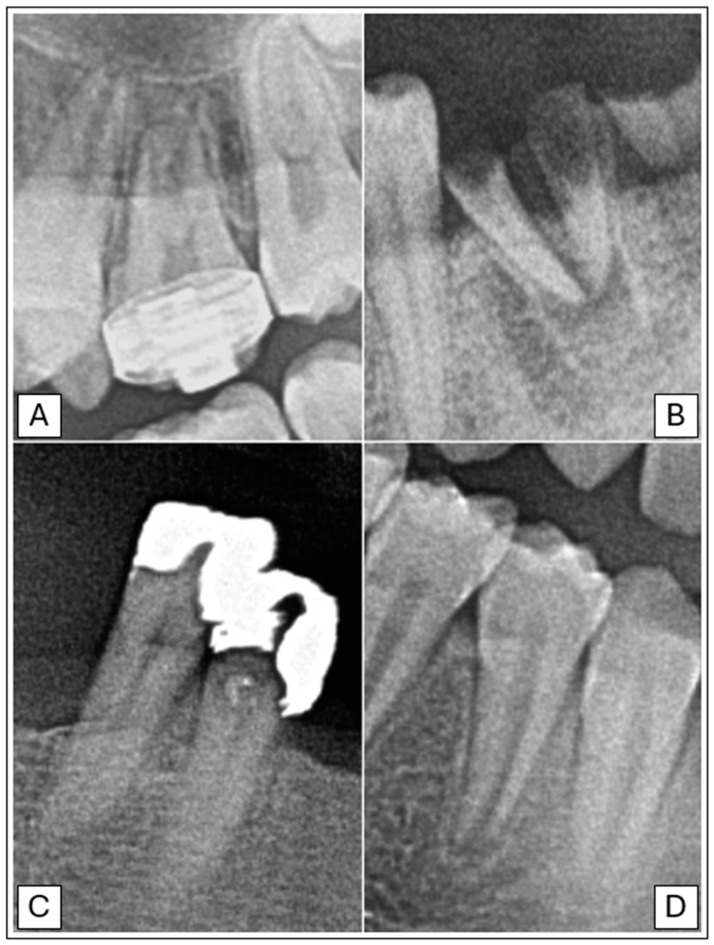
Examples of teeth misdiagnosed by AI software. (A) Tooth 26 with an orthodontic band, mistakenly identified as a tooth with a crown. (B) Tooth 36, not treated endodontically, incorrectly diagnosed as a tooth with root canal filling. (C) Teeth 44 and 45 with extensive, highly radiopaque dental fillings, wrongly identified as teeth with crowns. (D) Tooth 44 with incomplete root development, misdiagnosed as a tooth with a periapical lesion.
